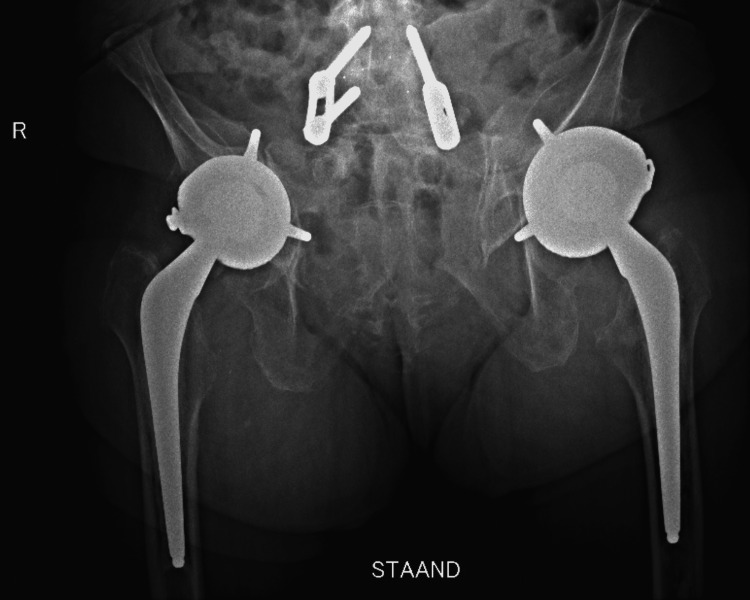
Pelvic x-ray showing bilateral total hip arthroplasty after acetabular revision surgery
